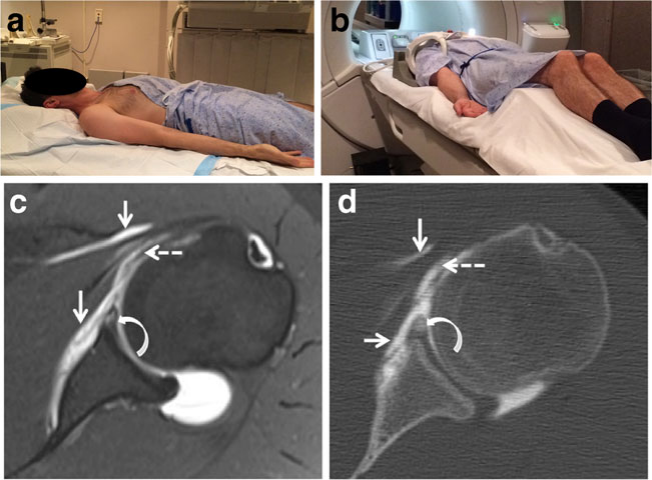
a, b Patient positioning. Patient is placed supine, head first, arm along the body, and the arm in external rotation for both fluoroscopy guided injection (a), and subsequent MR examination (b). c, d Drawbacks of the anteroinferomedial approach in shoulder CT arthrography and MR arthrography. Axial (c) fat-suppressed T1- and (d) correspondent CT - arthrography image show distortion of the subscapularis muscle with peri- (*straight arrows*) and intra-muscular (*dashed arrow*) contrast media extravasation caused by the needle traversing the subscapularis muscle. In addition, it is unclear whether intralabral contrast is also related to needle misplacement or actual tear (*curved arrow*). This can add difficulty to the interpretation. An anterior-rotator interval approach would have been optimal in this case
